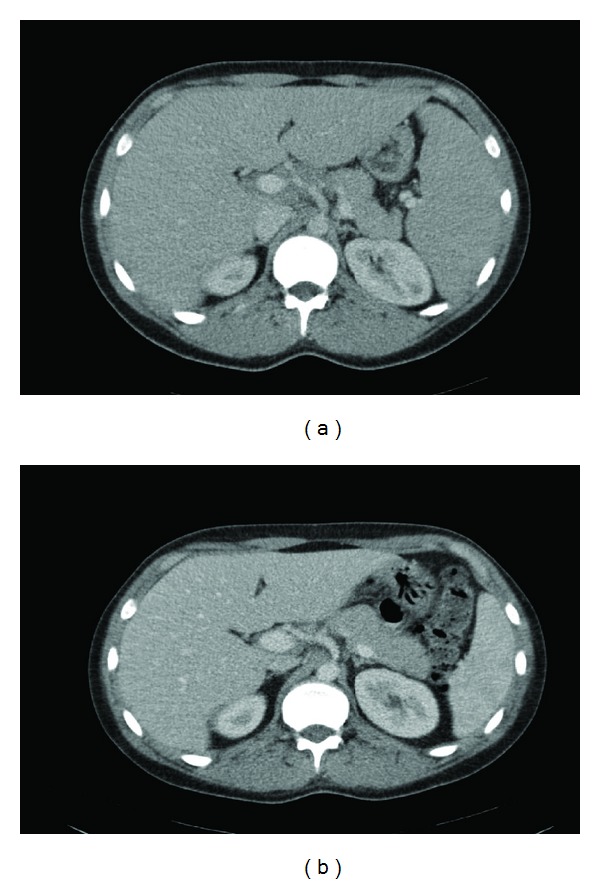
CT scan of the abdomen before (a) and after (b) first line of chemotherapy.
